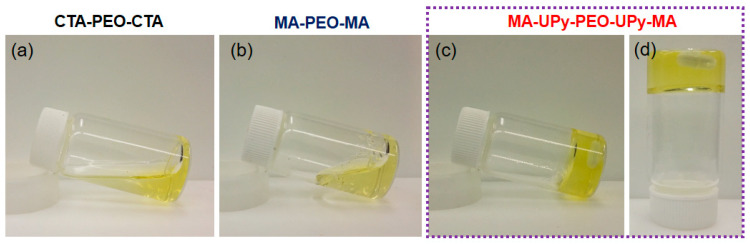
The gestation test of supramolecular hydrogel: (a,b) the solution of CTA-PEO-CTA and MA-PEO-MA is at a sol state at room temperature, while the solution of MA-UPy-PEO-UPy-MA can form a stable hydrogel (c,d).
